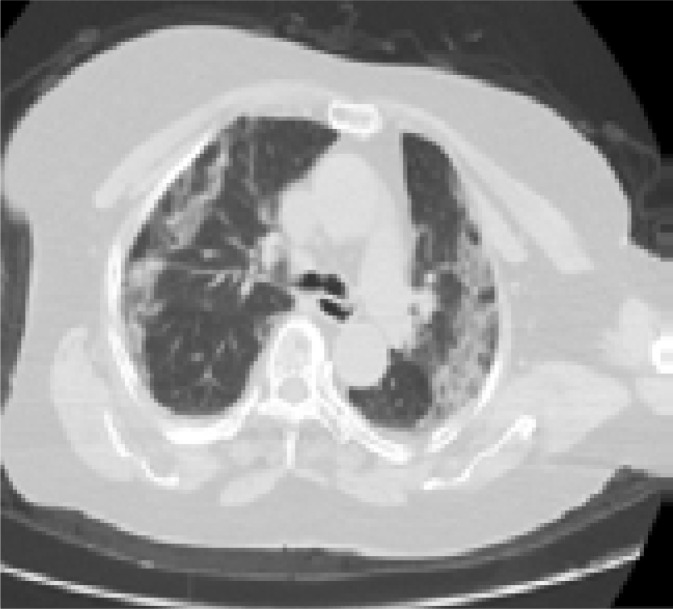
H) A 64-year old male patient with HT history had the complaints of fever, cough, and shortness of breath for 12 days. The patients applied due to progression of dyspnea for 2 days. Density increases of focal ground glass density, consolidations, and focal atelectasis appearances were found, more specifically in posterior segments and lower lobes of both lungs, suggesting a severe COVID-19 in chest CT image of the patient (They are indicated in black oval). The patient was intubated in our hospital and died in intensive care unit around 12 hours later.
